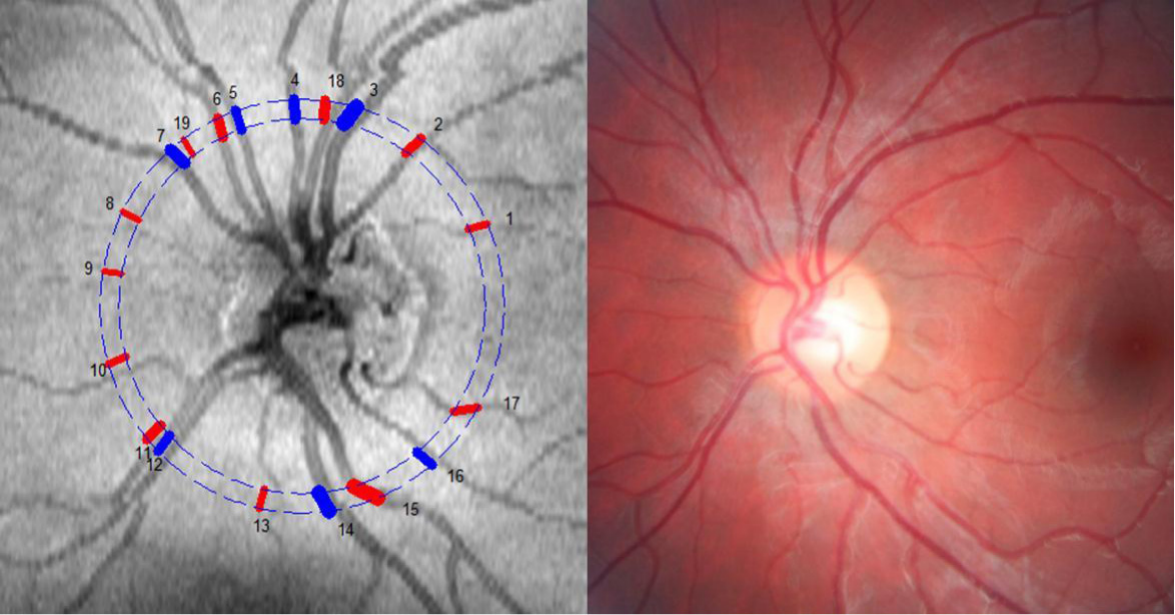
Left: Fundus image from Doppler FD‐OCT instrument for participant #9. The numbers indicate vessel location and the colors represent arterioles (red) or venules (blue). Right: Corresponding color fundus image for participant #9.
